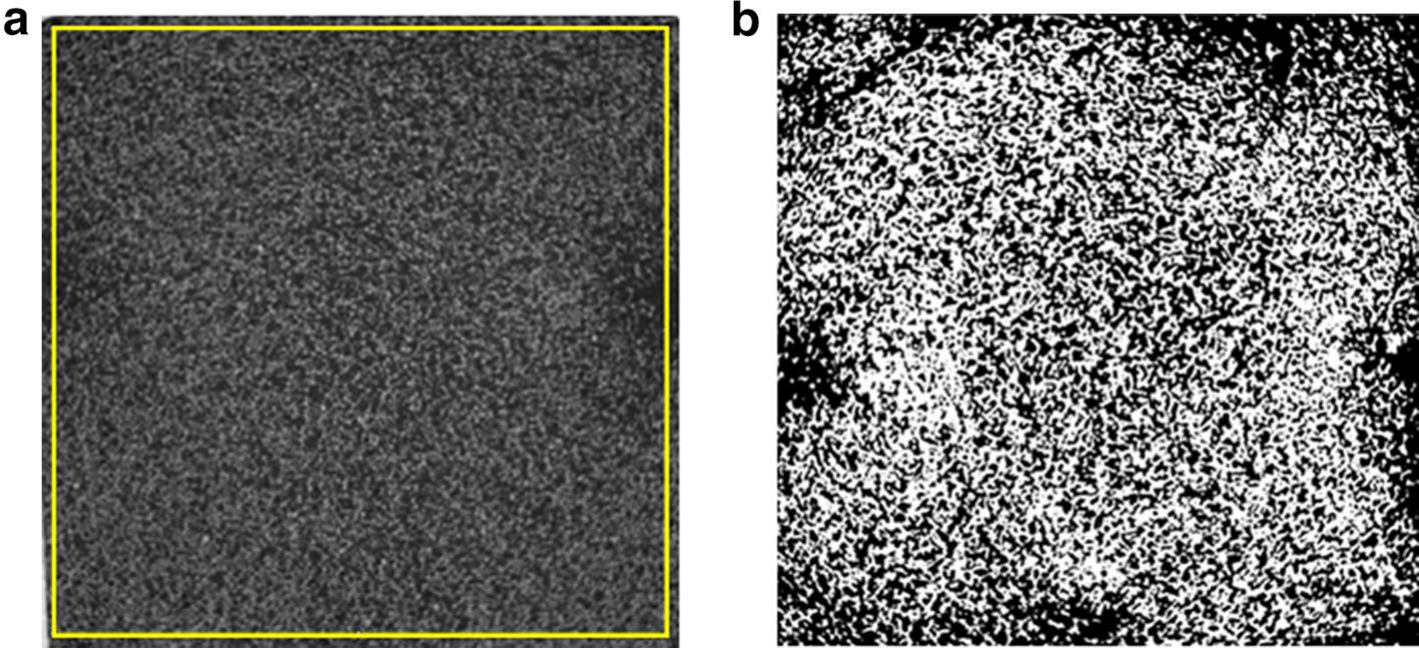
Example of 45 PPI foam structure along the *x*–*y* axis captured using CT where: (a) pre- and (b) post-processed data. Yellow outline indicates area of crop extraction to ensure no artefacts from the Perspex mould.
